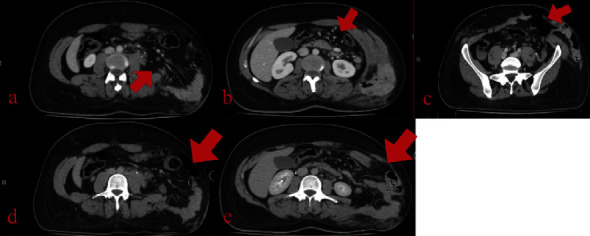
CT abdomen-pelvis imaging: (a–e) axial slices, demonstrating the TAWH containing both small and large bowels.
